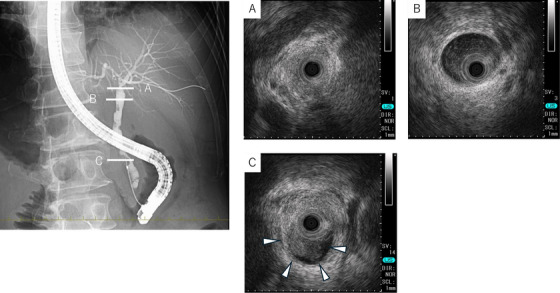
Endoscopic retrograde cholangiopancreatography (ERCP) revealed multiple intraductal protrusions without frayed, beaded, or diverticulum‐like features. Biopsies were taken from the sites labeled A and C. Representative intraductal ultrasonography (IDUS) images are shown: (A) Hilar bile duct: Circumferential luminal narrowing was evident, with disruption of the outer hyperechoic band and loss of the normal layered architecture. (B) Non‐stenotic region: The bile duct wall was smooth, with preservation of both the layered structure and the outer hyperechoic band. (C) Distal bile duct: A protruding lesion was visualized at the 8 o'clock position.
